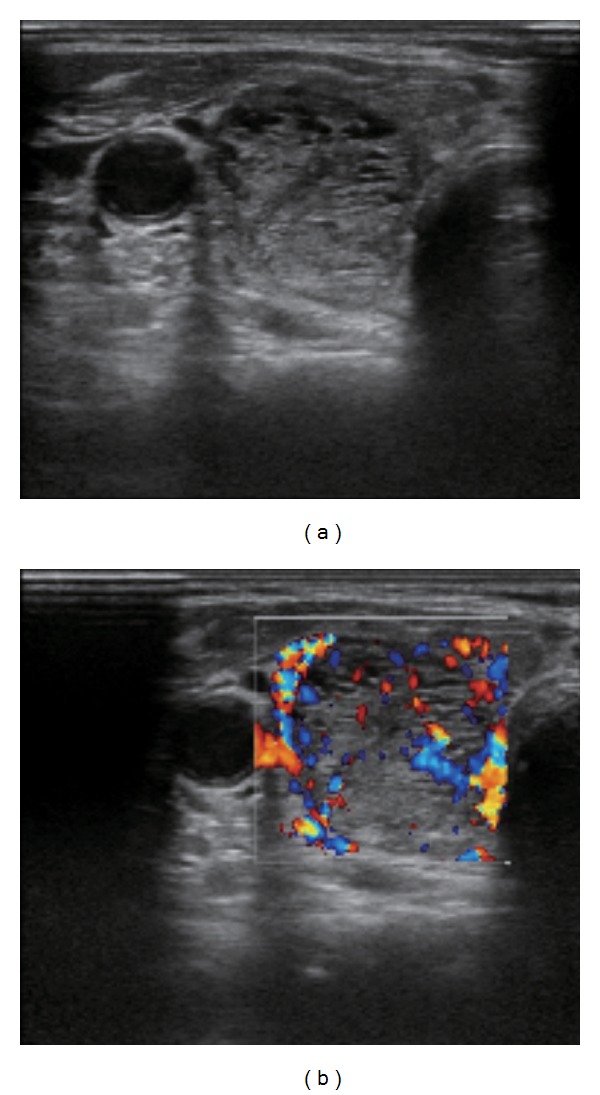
Ultrasonography of the right lobe. A hypoechoic, inhomogeneous mass with a sharp border was detected in the right lobe. The tumor measured 21.5 mm × 19.4 mm × 36.3 mm in diameter. Doppler color flow revealed a high vascularity of the tumor.
